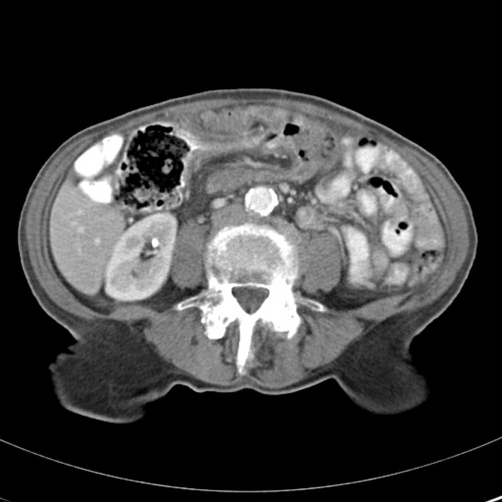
CT Scan abdomen with evidence of intussusception at the transverse colon.
